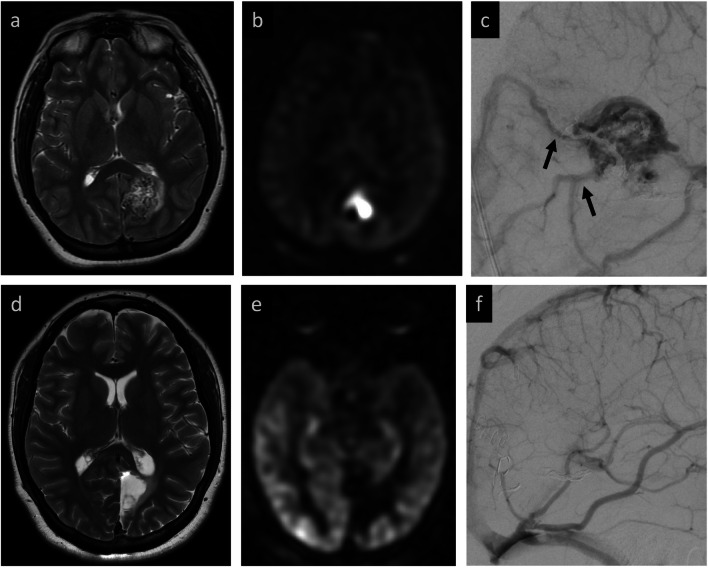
Concordant ASL and DSA findings confirm the absence of residual AV shunting after surgical resection. Baseline MRI: tangle of vessels in the left occipital lobe reflects the AVM nidus (a), with corresponding marked ASL signal (b) secondary to shunting. Baseline DSA: lateral projection demonstrates the AVM with draining veins extending posteriorly from the nidus (black arrows, c). Note nidal embolization material from prior treatment. Post-resection MRI: fluid-filled resection cavity without visible tangle of vessels (d) or elevated ASL signal to suggest residual shunting (e). Post-resection DSA: concordant findings of no residual AVM (f)
